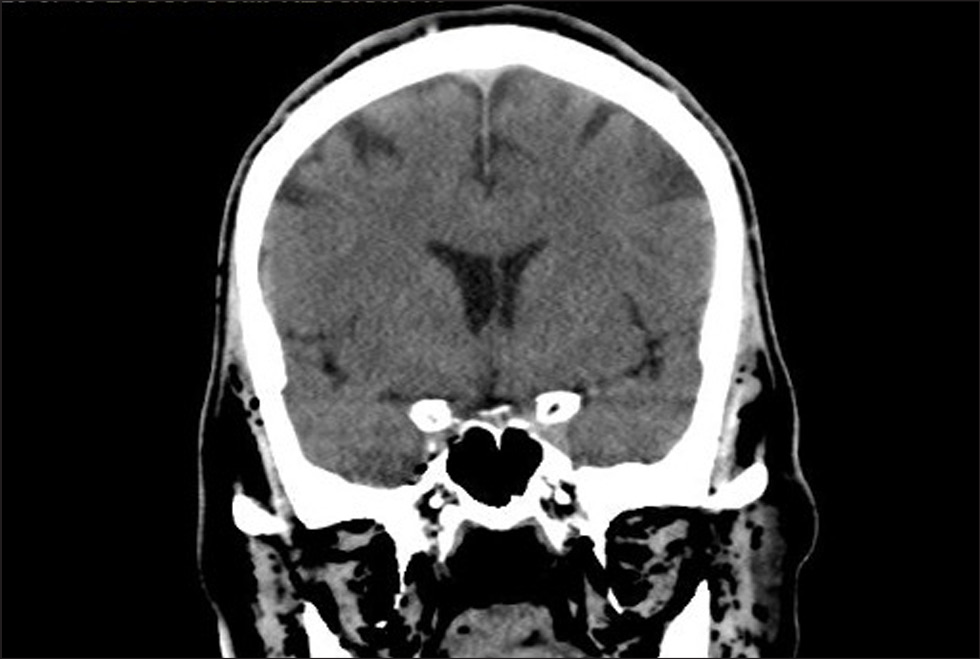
Computed tomography of the head revealing the presence of air in facial tissue.
